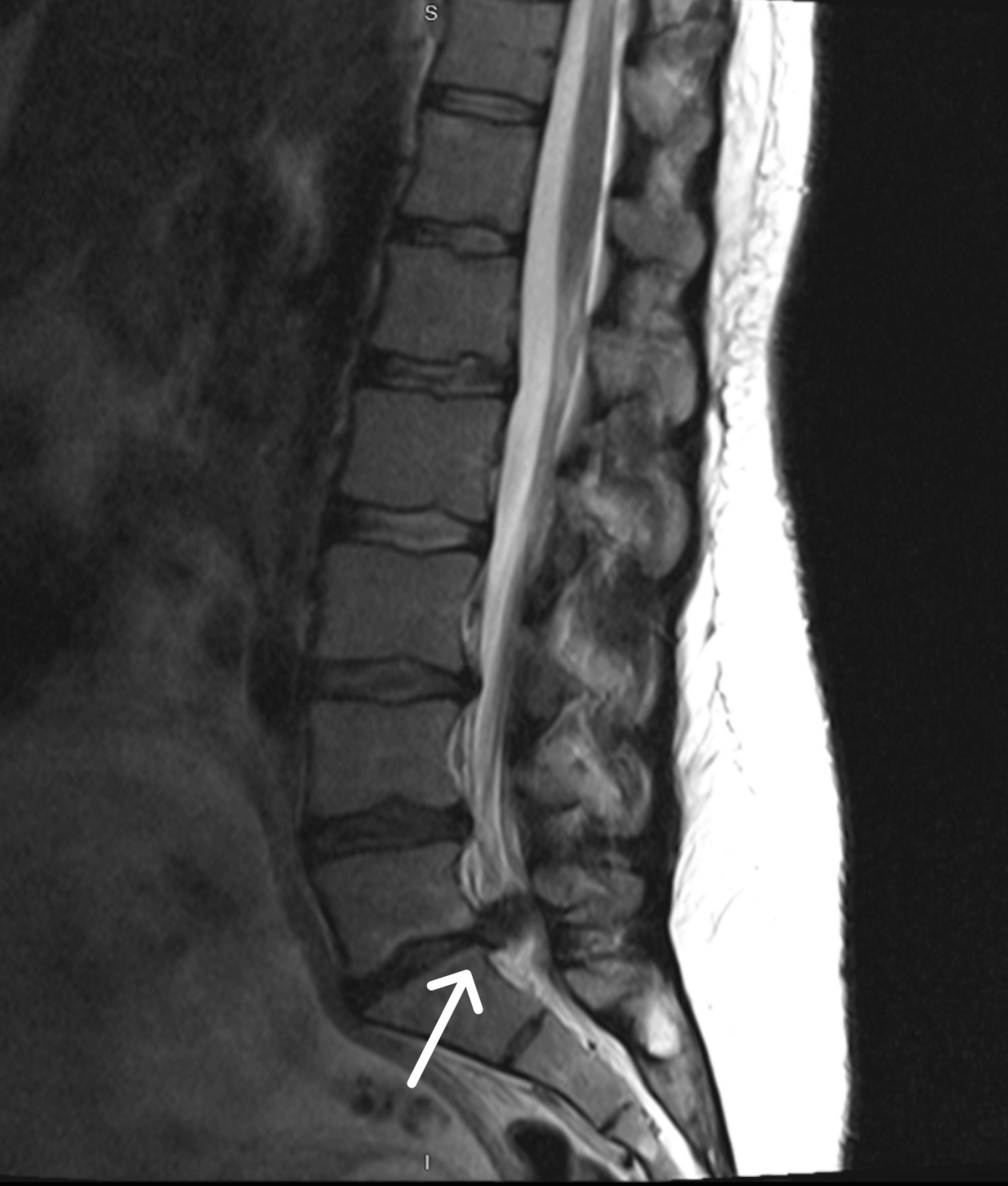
MRI Lumbar Spine: T2-weighted sagittal view demonstrating large L5/S1 disc protrusion/extrusion
